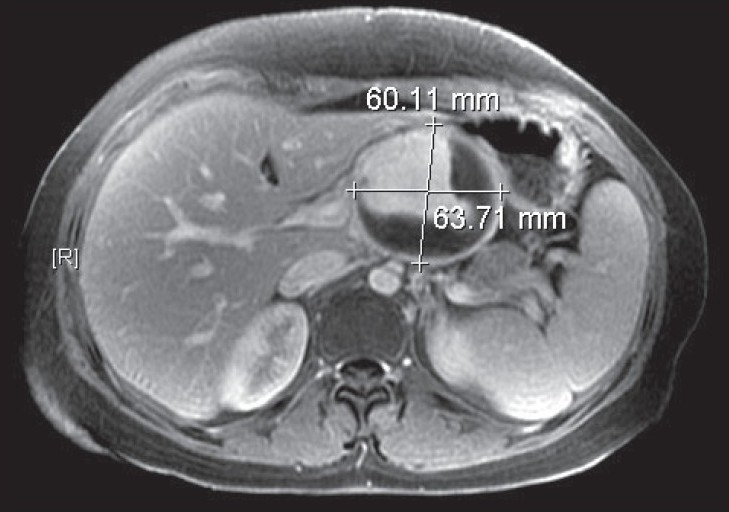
Magnetic resonance imaging of the abdomen demonstrates a large celiac artery aneurysm involving the trifurcation
